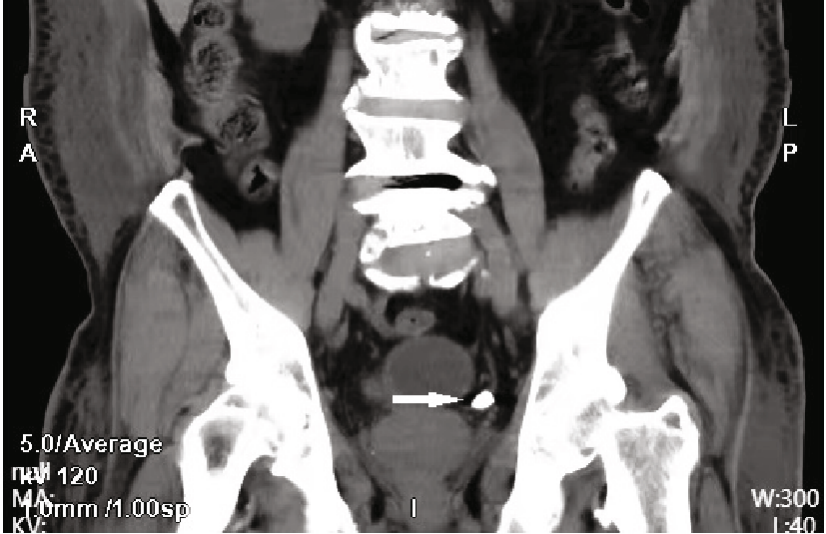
CT image on coronal view in 2023 shows a pelvic stone near the bladder (white arrow).
